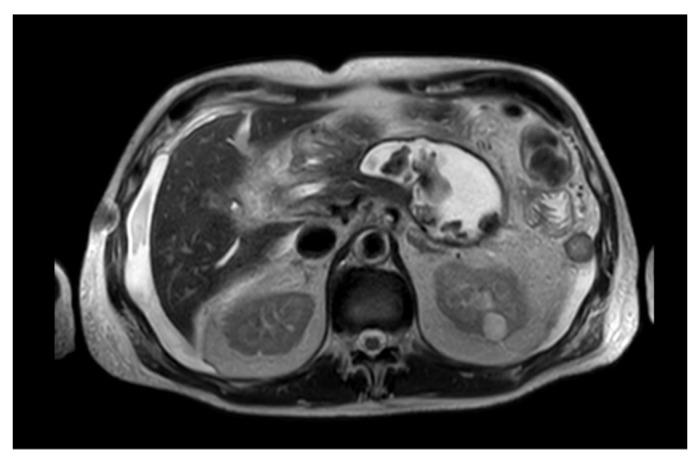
MRI image of a WOPN with both liquid and solid necrotic material within.
